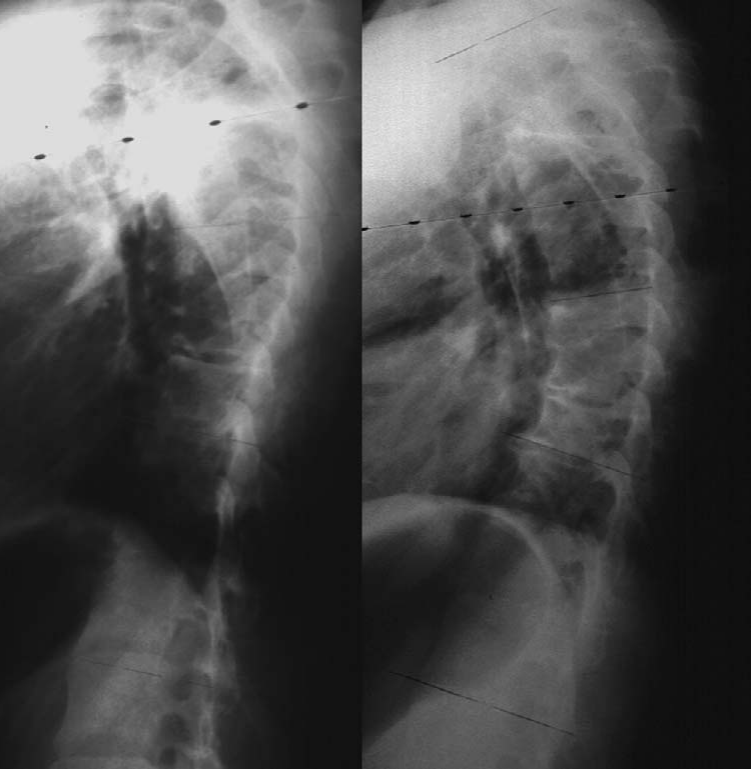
Multiple vertebral wedge osteotomy. Lateral radiographs before and after surgery.
